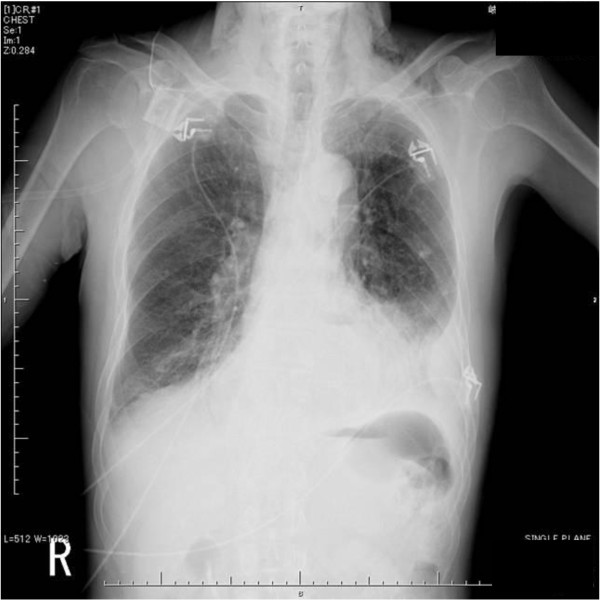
Plain chest X-ray radiography. No apparent free air was present, but marked right pleural effusion was observed.
